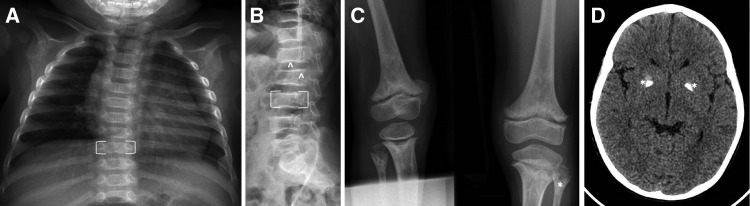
Radiological features of SPENCD. (A) Chest X ray showing platyspondyly (representative example indicated by the open brackets) present in a 4-mo-old male homozygous for a c.643G>A (p.(Gly215Arg)) mutation in *ACP5*. (B) Lateral spinal X ray showing platyspondyly (representative example indicated by the open brackets) and irregular vertebral margins (representative example indicated by: ^) in a 10-year-old male homozygous for a c.821T>C (p.(Val247Ala)) mutation in *ACP5*. (C) X ray of femur, tibia, and fibula showing metaphyseal enchondroma (representative example in the left fibula indicated by: *), particularly involving the tibia and fibula of an 11-year-old male homozygous for a c.667C>T (p.(Gln223*)) mutation in *ACP5*. (D) Cranial CT scan showing bilateral basal ganglia calcification (indicated by: *) in the same child as seen in C at the age of 9 years.
